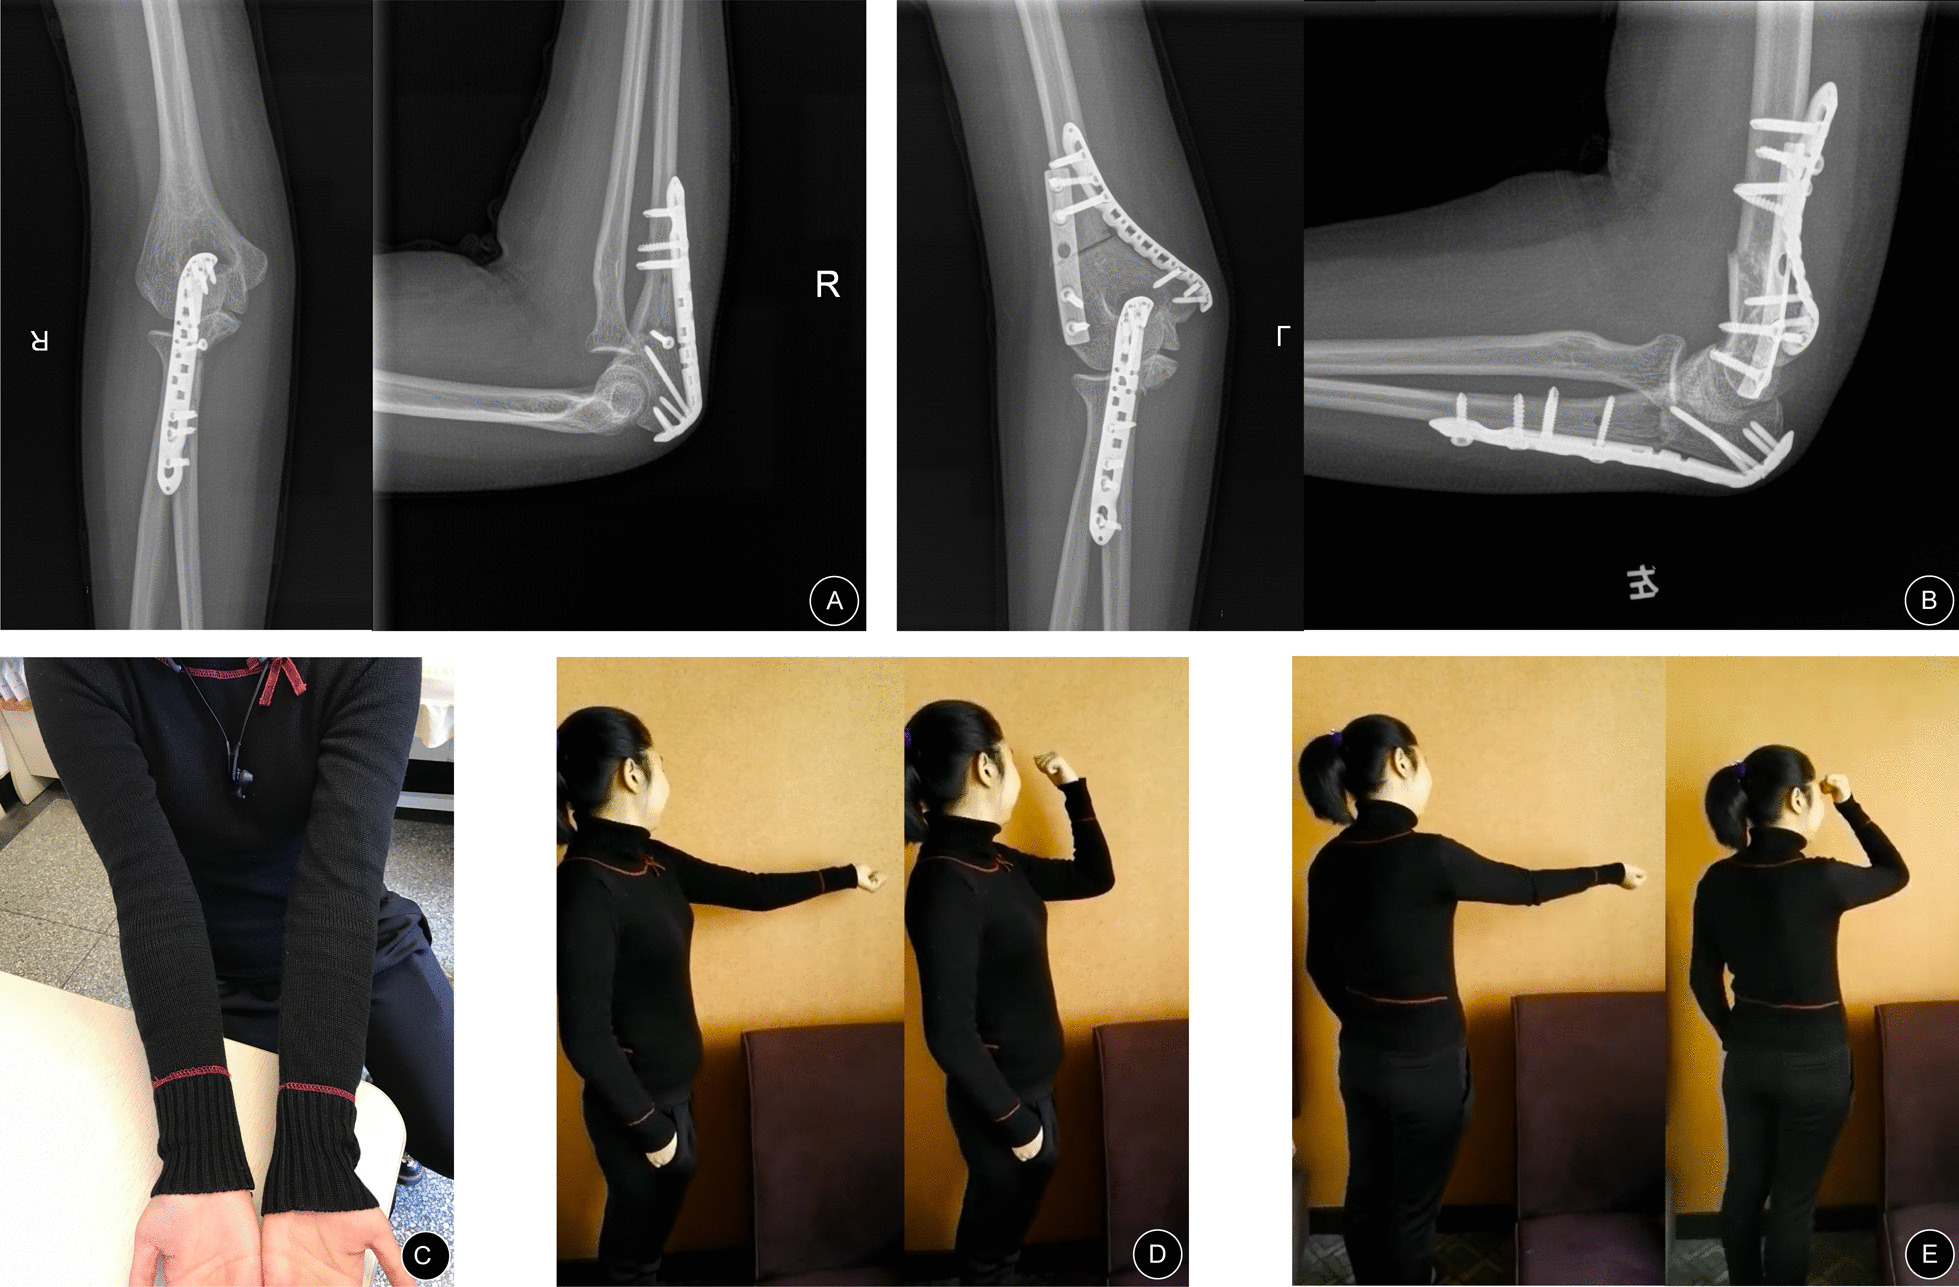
A 19-year-old woman presented with bilateral cubitus valgus deformities after surgery (Case 11): A, B Appropriate corrections were achieved as evident on the postoperative anteroposterior and lateral radiographs. C The valgus deformities were corrected. D, E The range of flexion and extension of the bilateral elbow joints after surgery was good (Left, 20°–120°; Right, 20°–130°)
